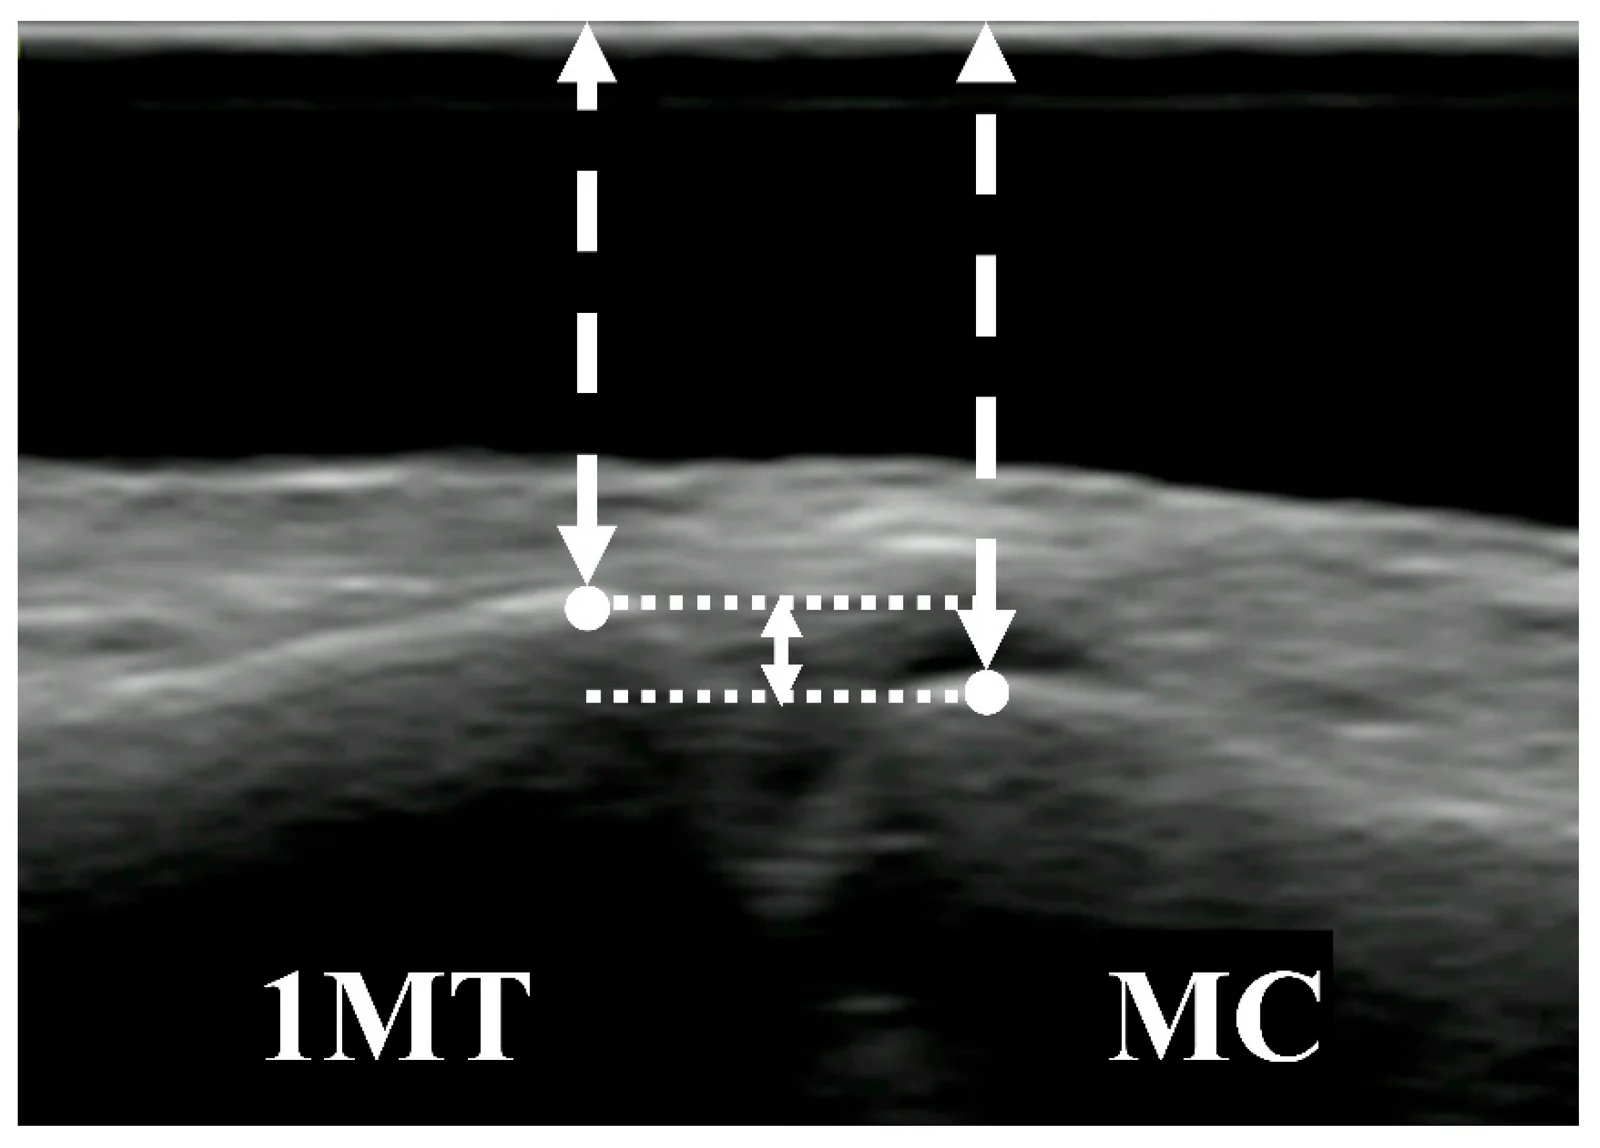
Representative ultrasonographic image of the first tarsometatarsal (TMT) joint. The dorsal cortices of the first metatarsal (1MT) and medial cuneiform (MC) are visualized. The vertical position of the 1MT and MC were defined as the perpendicular distance from the superior border of the image to the dorsal cortex of each bone (long dashed double-headed arrow). The first TMT joint gap was calculated as the difference between these vertical distances (solid double-headed arrow).
